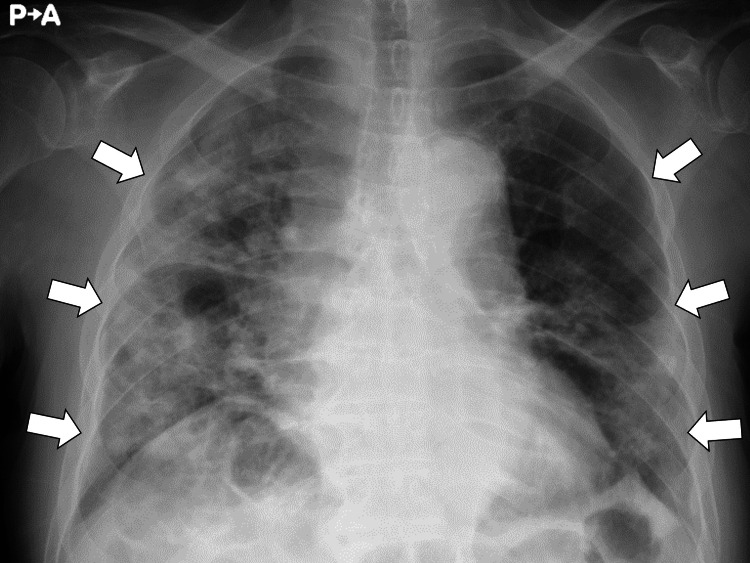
Chest radiography demonstrating bilateral subpleural infiltrative shadows (white arrows)
